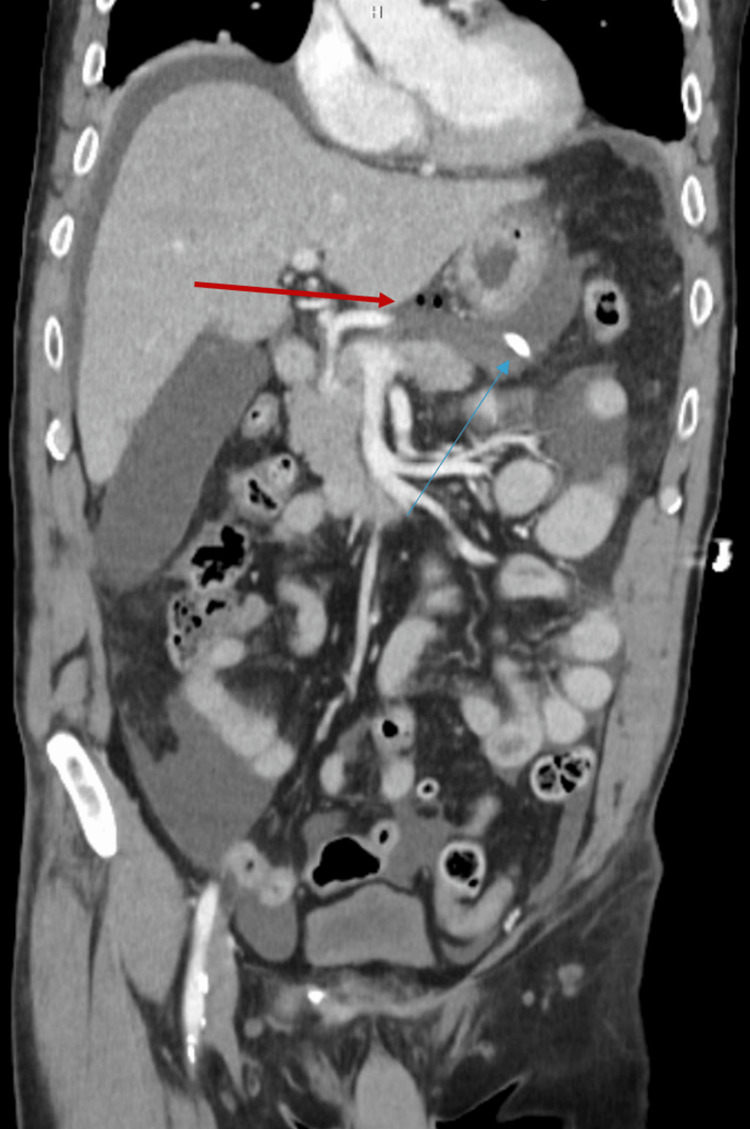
Coronal computed tomography image demonstrating the nasogastric tube extending from the distal esophagus across the gastroesophageal junction into the proximal gastric wall (blue arrow) with associated free air (red arrow).
